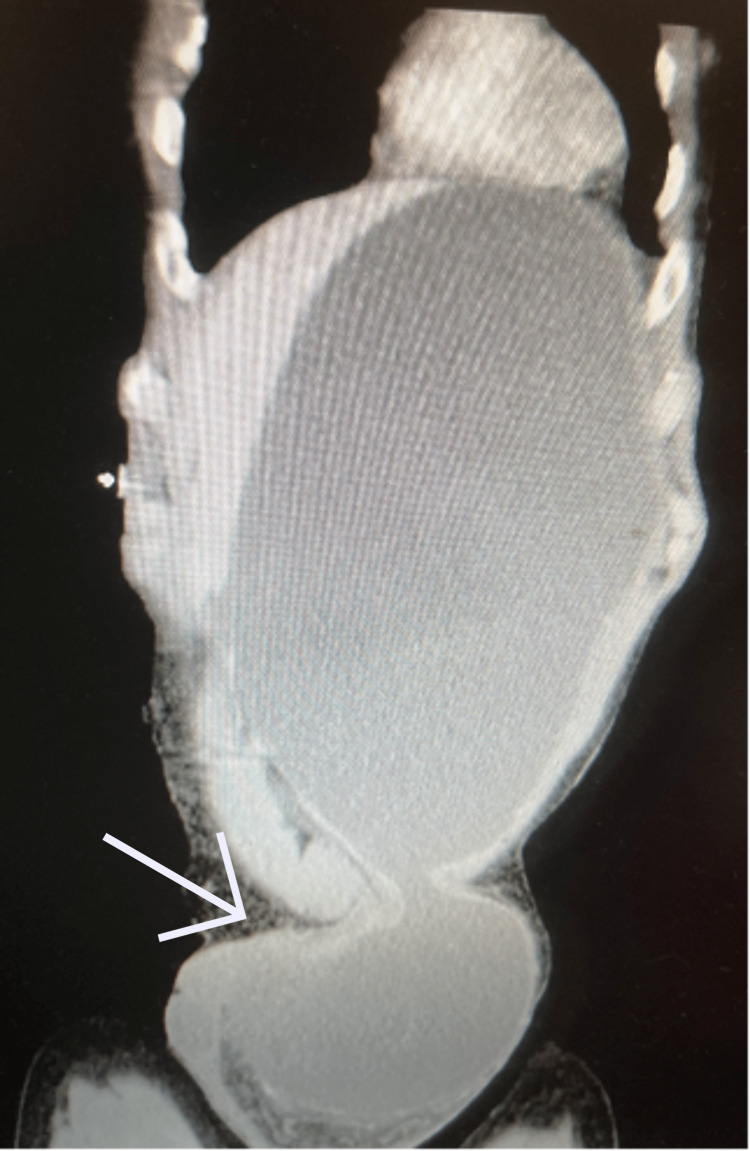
Computed tomography of abdomen and pelvis coronal cross section showing dilated stomach extending into the left inguinal hernia. Arrow pointing to incarcerated left inguinal hernia containing stomach.
